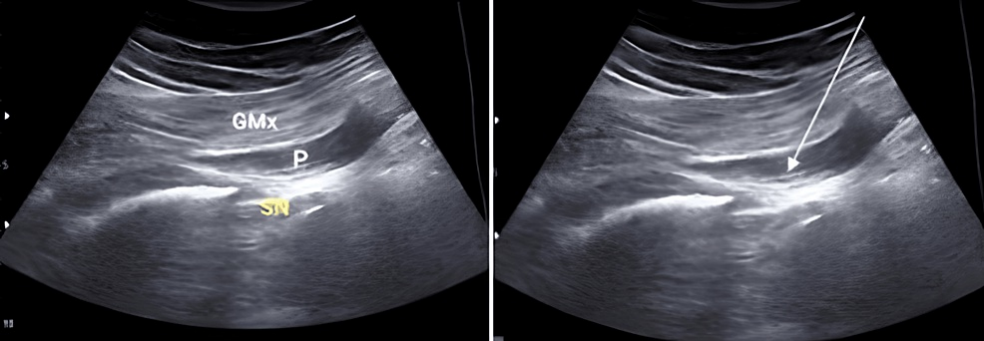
Ultrasound-guided piriformis muscle dry needling with in-plane technique from medial to lateral GMx: gluteus maximus muscle; P: piriformis muscle; SN: sciatic nerve; arrow: block needle.
